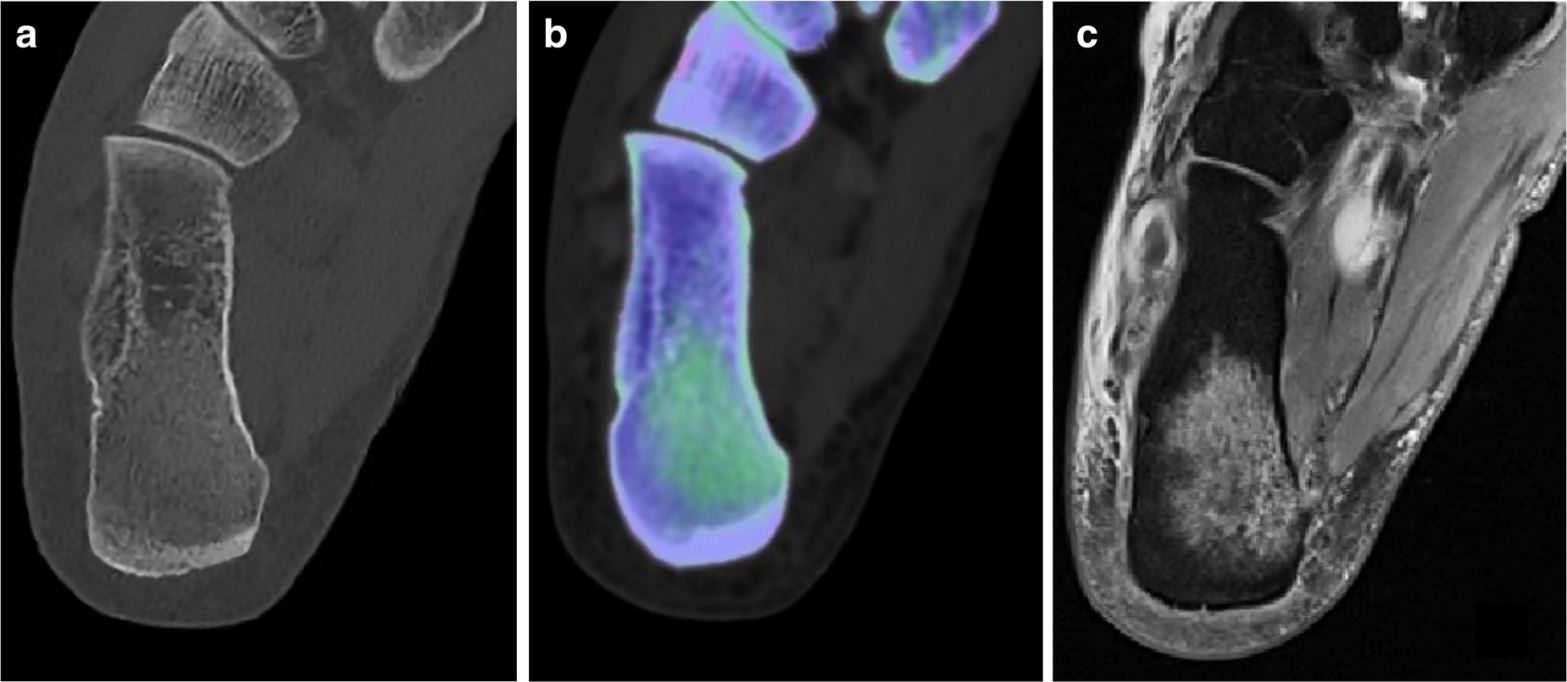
Dual-energy computed tomography scan in a patient presenting with right-sided acute ankle trauma. Standard grayscale series (a) does not depict any fracture line. Color-coded virtual non-calcium image (b) shows a distinct traumatic bone marrow edema of the right calcaneus, displayed as a green area (*arrow*). The finding was confirmed by magnetic resonance imaging (c) using a proton density-weighted sequence (*arrow*)
